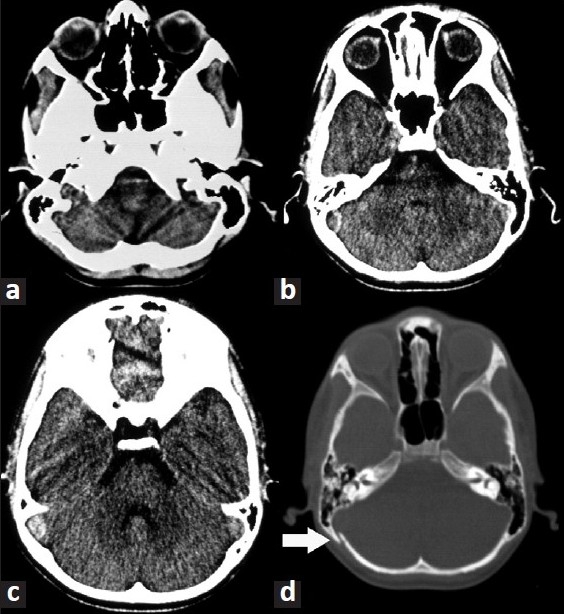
CT scan (a–d) showing hyperdense area in the region of right sigmoid sinus and sutural diastases of right lambdoid suture (arrow in D).
